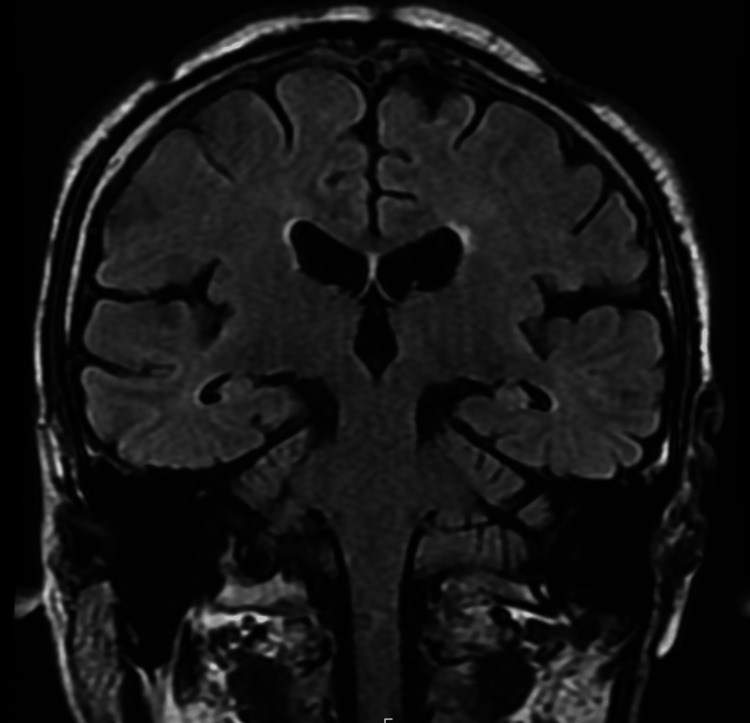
Brain MRI (FLAIR imaging) FLAIR imaging shows no evidence of chronic ischemic changes in the brainstem. MRI: magnetic resonance imaging, FLAIR: fluid-attenuated inversion recovery
